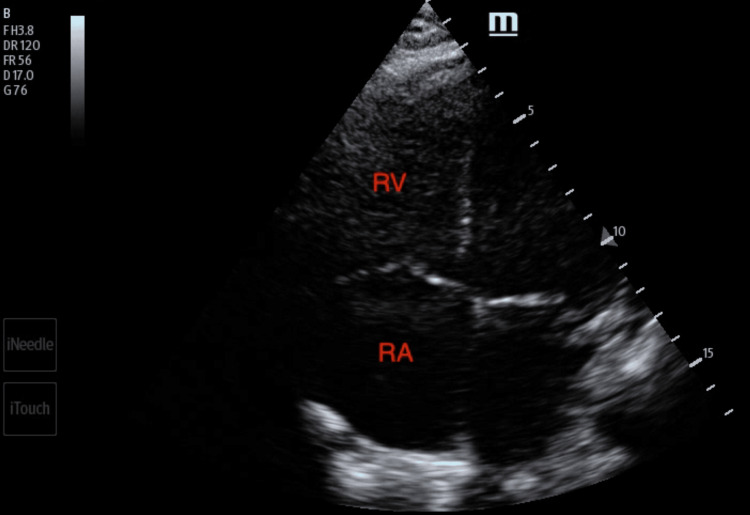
Transthoracic echocardiogram done in the apical four-chamber view post-thrombolysis, showing complete resolution of the thrombus previously visualized in the right atrium. RA: right atrium; RV: right ventricle
